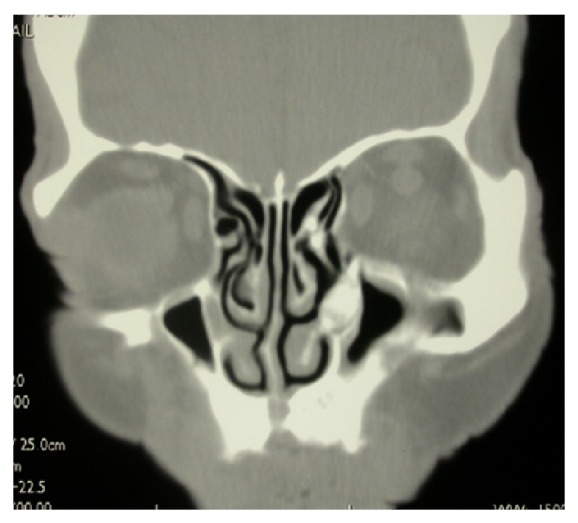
Coronal view of the CT scan of the paranasal sinus showing the osteoma of the medial wall of the left-sided maxillary sinus.
